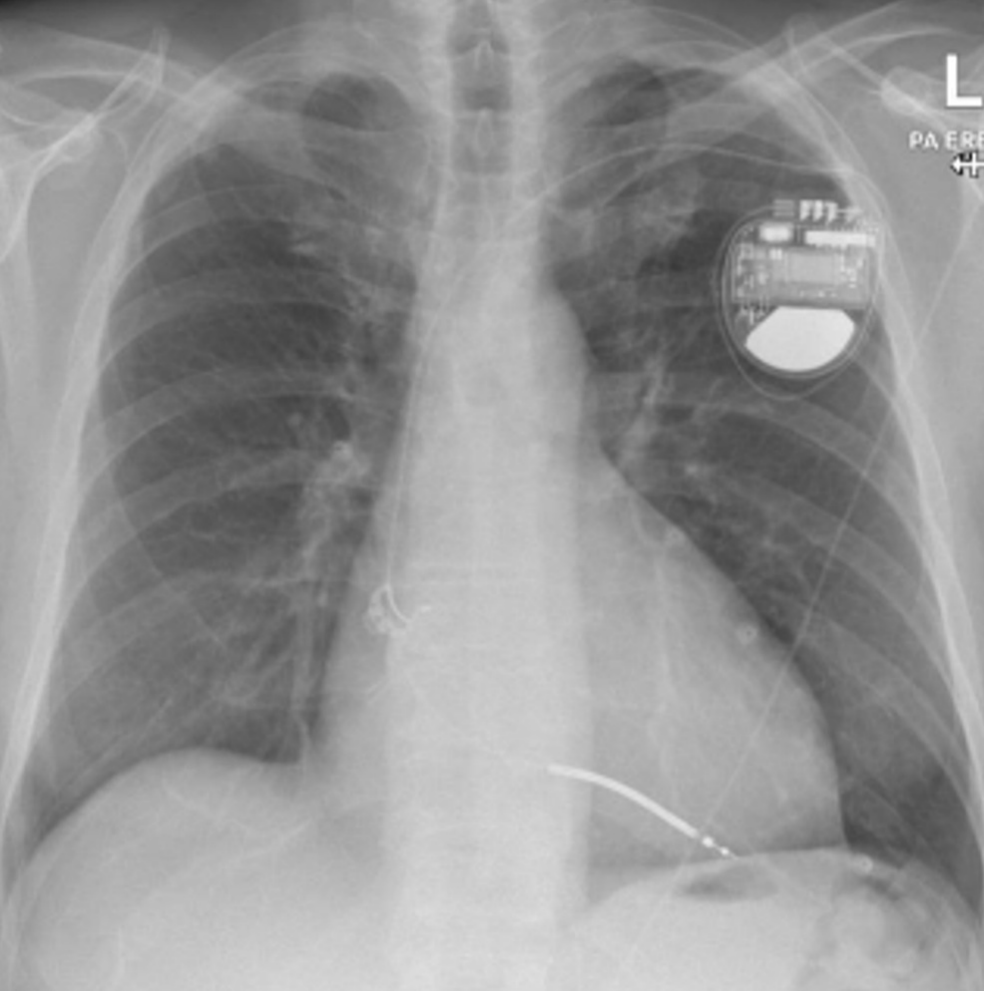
Chest X-ray showing single-chamber implantable cardioverter-defibrillator (ICD)
